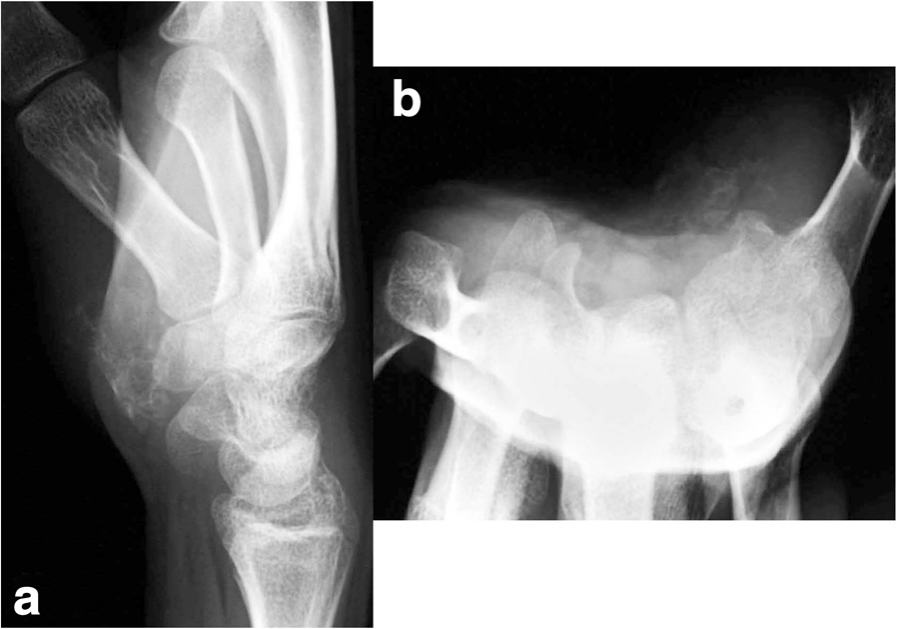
Radiographs of the case. (a) Lateral and (b) carpal tunnel aspects of the right hand showing ossification of soft tissues over the volar aspect of the thenar region.
